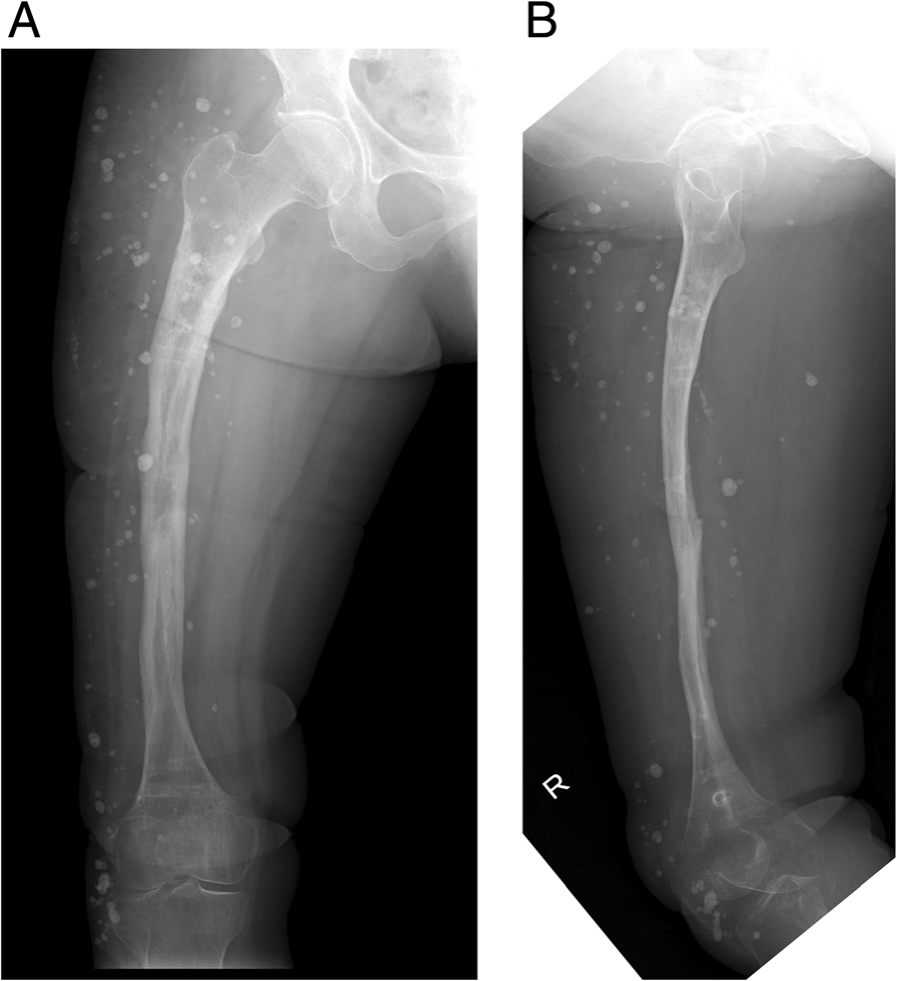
Anterolateral (a) and lateral (b) radiographs taken 24 months after the second surgery show complete bony union. The Taylor Spatial Frame was removed 11 months after the second surgery
